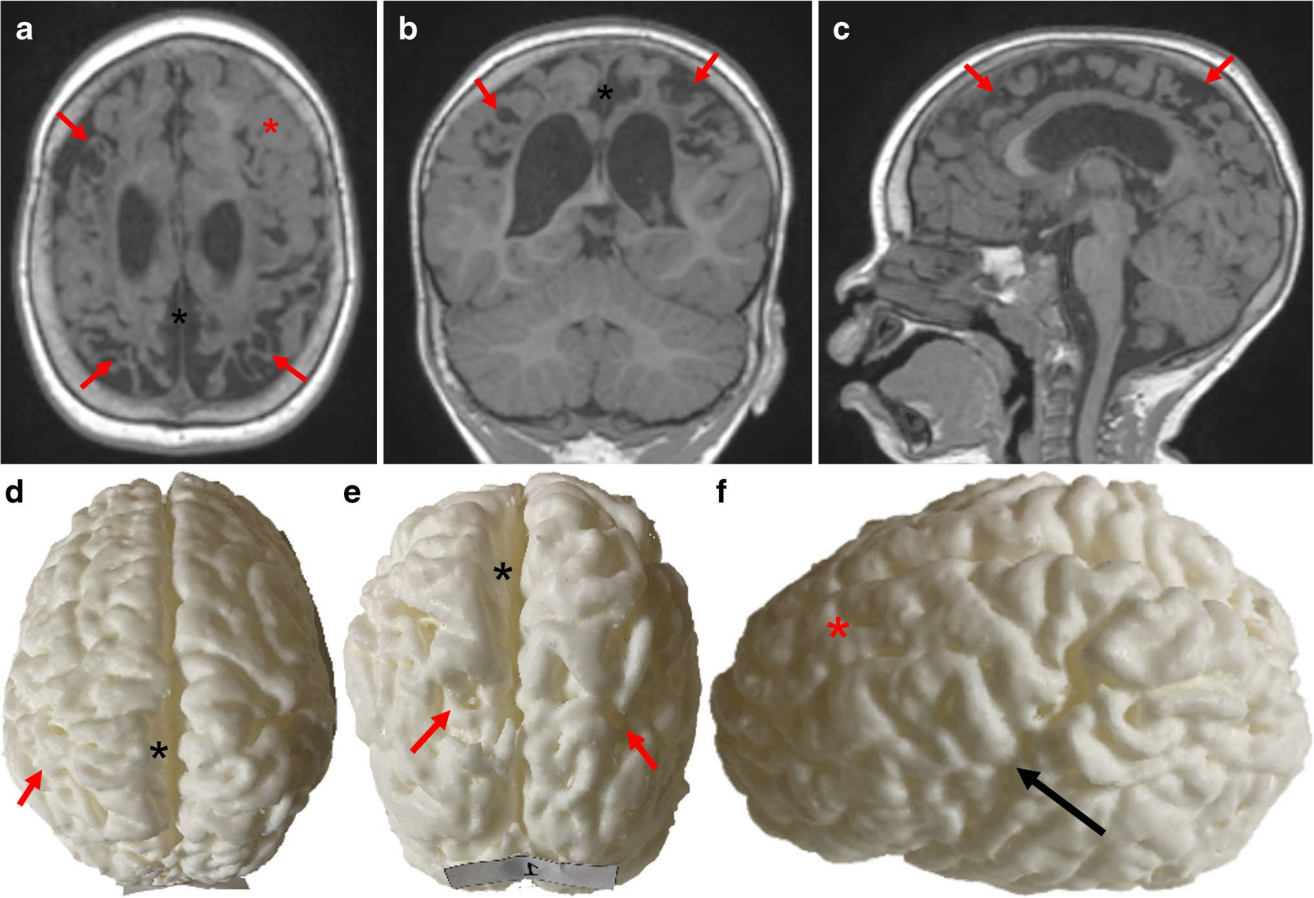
Images of a 2-year-2-month-old boy with cerebral palsy due to term partial prolonged hypoxic ischemia. a–c Axial (a), coronal (b) and sagittal (c) T1-weighted magnetic resonance images of the brain and (d–f) vertex (d), posterior (e) and left (f) side views of the corresponding 3-dimensional (D) printed model of the brain. Images show areas of injury with cortical atrophy (*red arrows*) clearly appreciated on the 3-D models, lentiform separation of the cerebral hemispheres (*black asterisks*) due to cerebral atrophy and volume loss in the parasagittal watershed regions, widening of the Sylvian fissure (*black arrow*) due cerebral atrophy and volume loss and relative sparing of the left anterior intervascular watershed (*red asterisk*)
